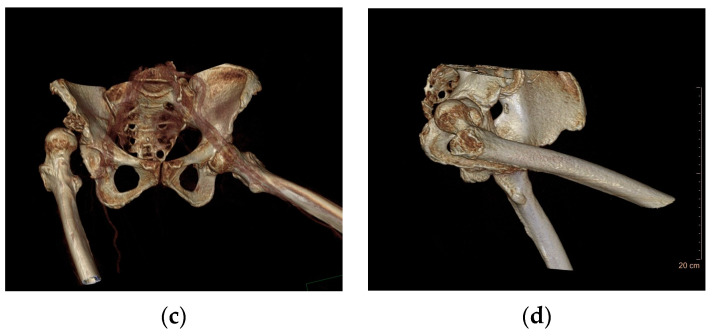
(a) Initial pelvic radiograph in which posterosuperior hip subluxation is observed without fractures; (b) Computerized Tomography (CT) scan (transverse). There is no presence of avascular necrosis of the right hip. (c,d) 3D reconstruction from the CT scan.
